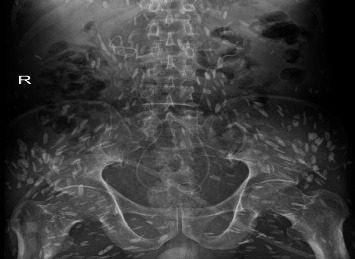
Kidney-Ureter-Bladder (KUB) radiograph, after ureteral stent placement.
